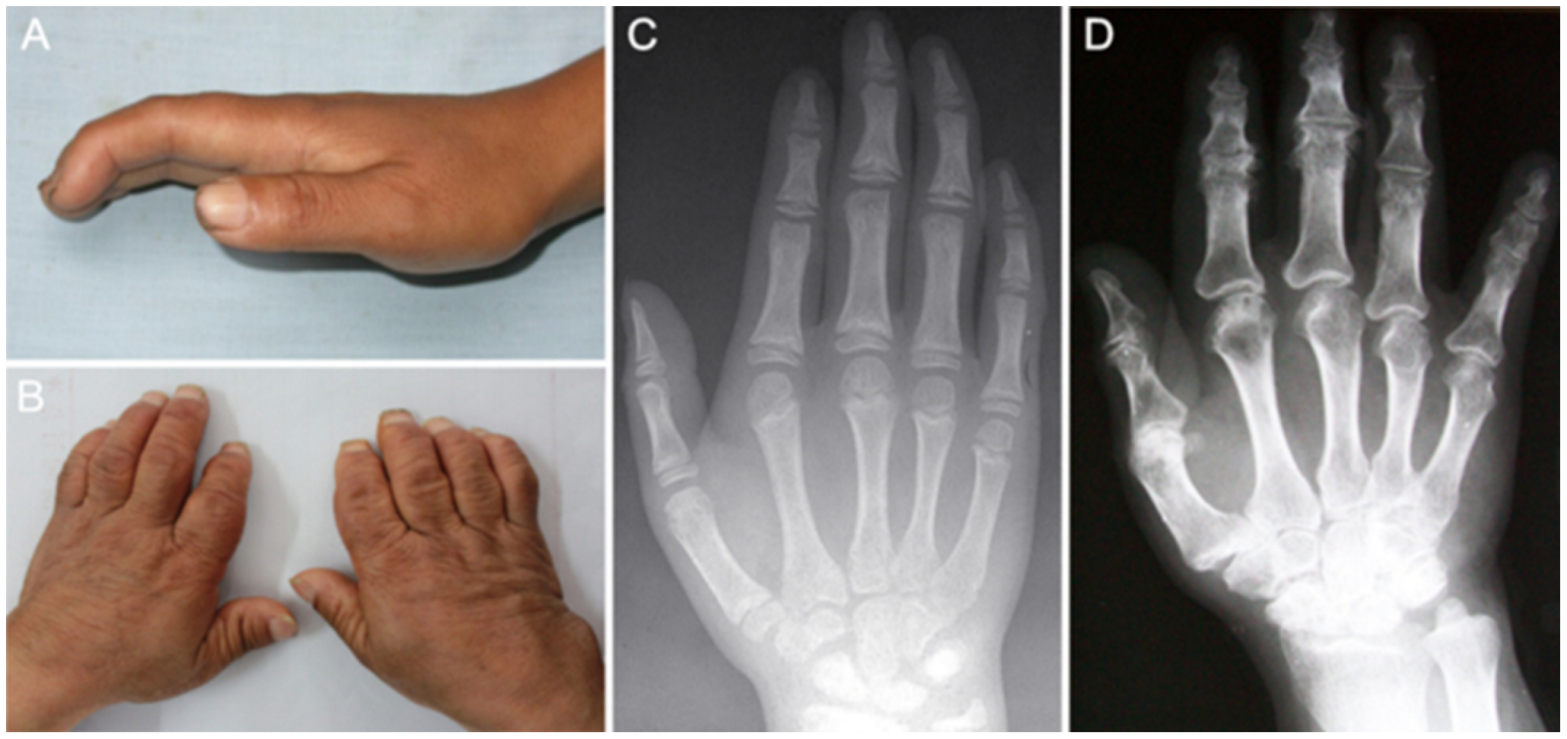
Characteristics of child and adult Kashin-Beck disease (KBD) patients. A, C: Image and radiograph of the left hand of a representative KBD child with grade I KBD (male, 12 years old). B, D: Image and radiograph of hands of a representative KBD adult with grade II KBD (female, 55 years old).
